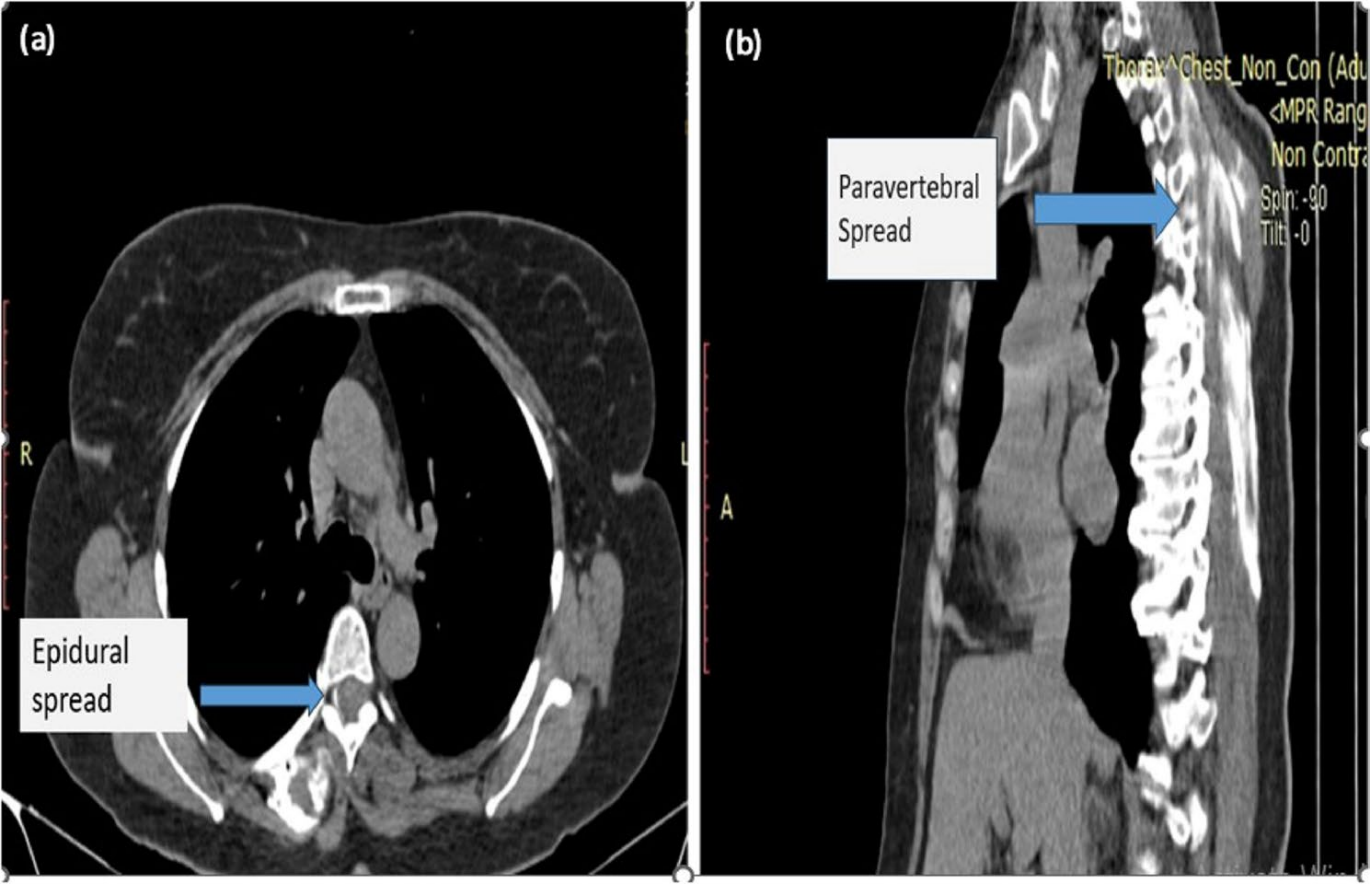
Computed Tomography scan demonstrating the paravertebral and epidural contrast spread after ESPB. (a) An axial (transverse) cut of the chest highlights Epidural Spread (A blue arrow). (b) A sagittal cut identifies Paravertebral Spread (blue arrow)
